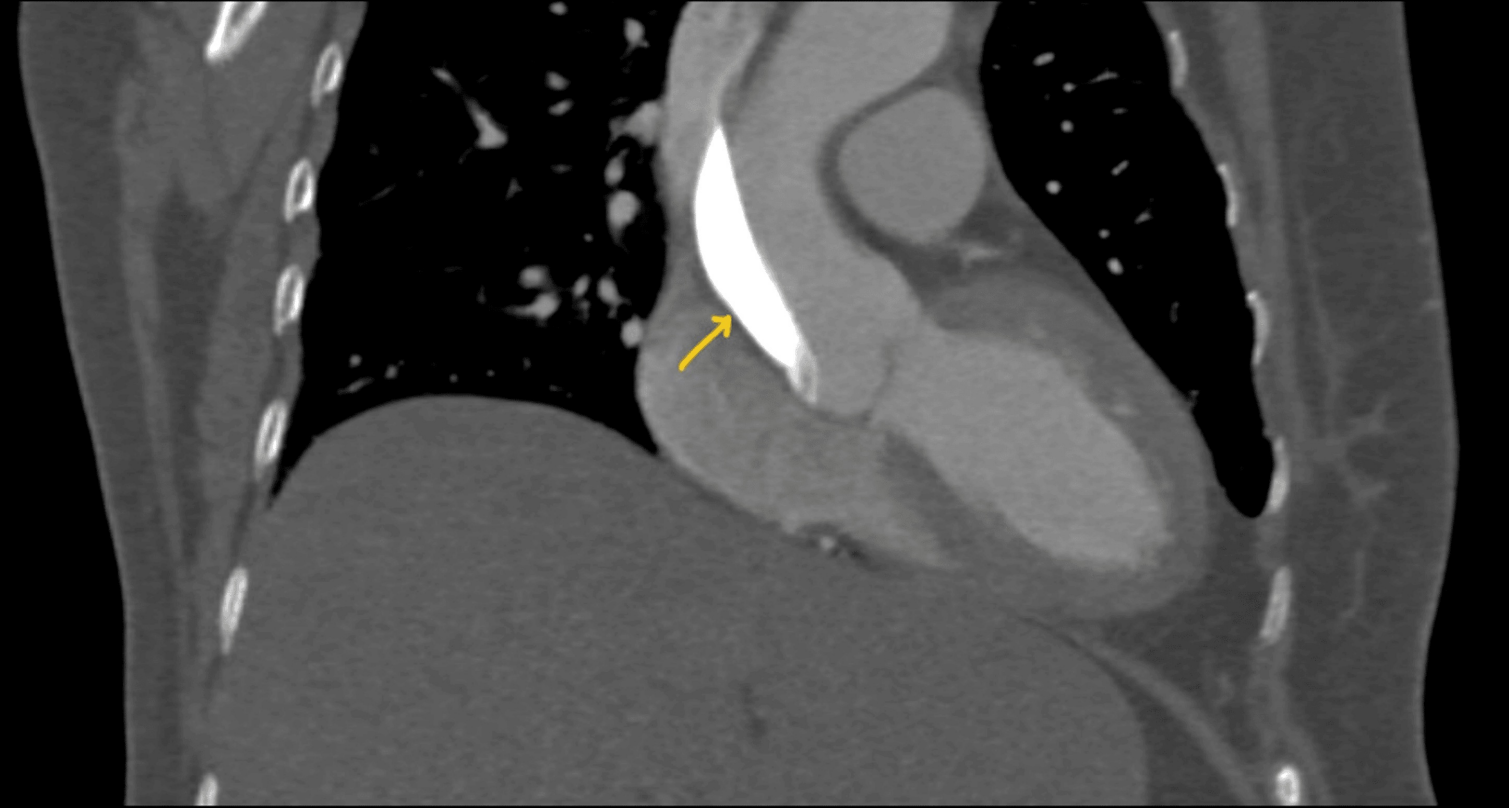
CT aortogram coronal section. Arrow indicates the further extension of contrast into dissection flap.
